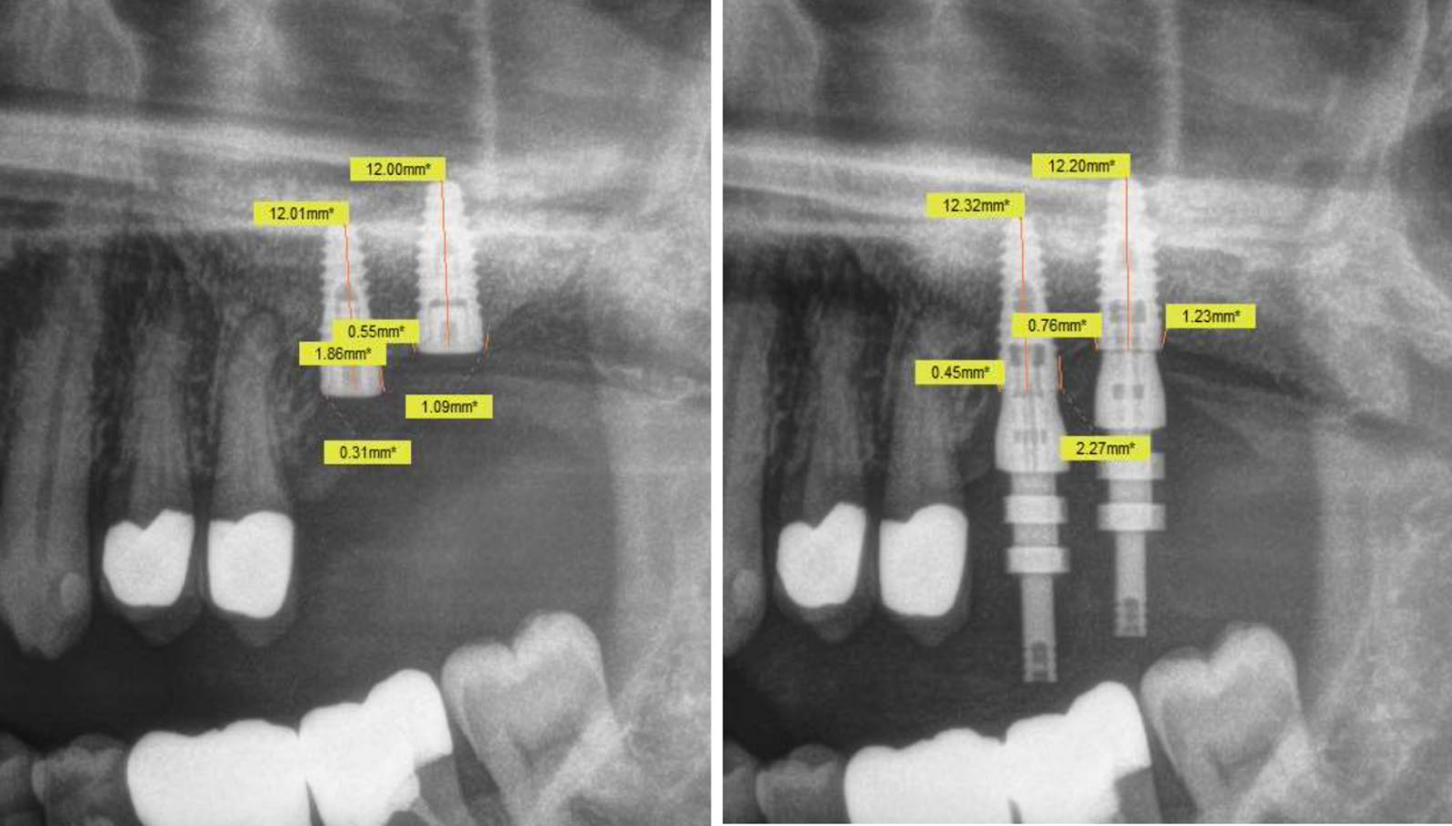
Analysis of radiographs using the measuring tool (Sidexis 4, Dentsply Sirona, York, USA) taken at the time of implantation (left) and after 6 months (right). The measuring tool was used to calculate distances between implant shoulder and marginal bone mesially and distally of the implant
